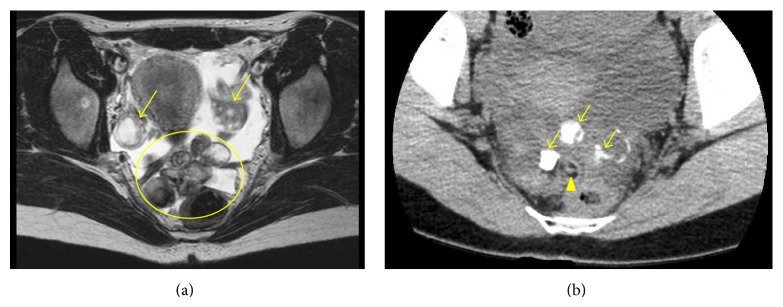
(a) T2 weighted magnetic resonance imaging showing a multicystic mass (circle) and normal ovaries (arrows). (b) Computed tomography showing calcified (arrows) and fatty (arrow head) components in the mass.
